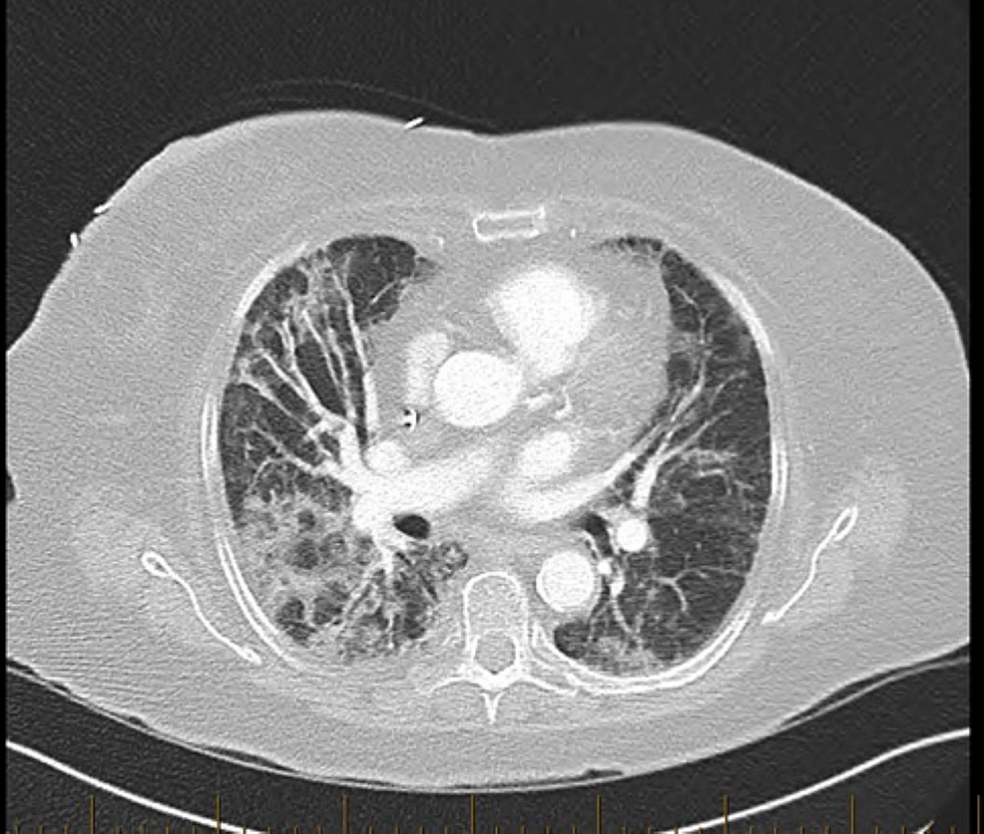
Computed tomography angiography (CTA) of the chest taken on the day of admission showing bilateral diffuse parenchymal opacities right greater than left with interlobular septal thickening and some mild central bronchiectasis.
